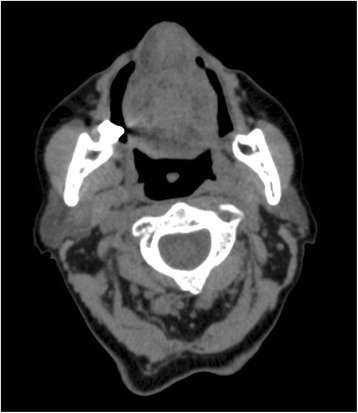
CT scan after 6 months of chemotherapy showing the complete disappearance of the tumor mass primarily located in the body of the tongue
